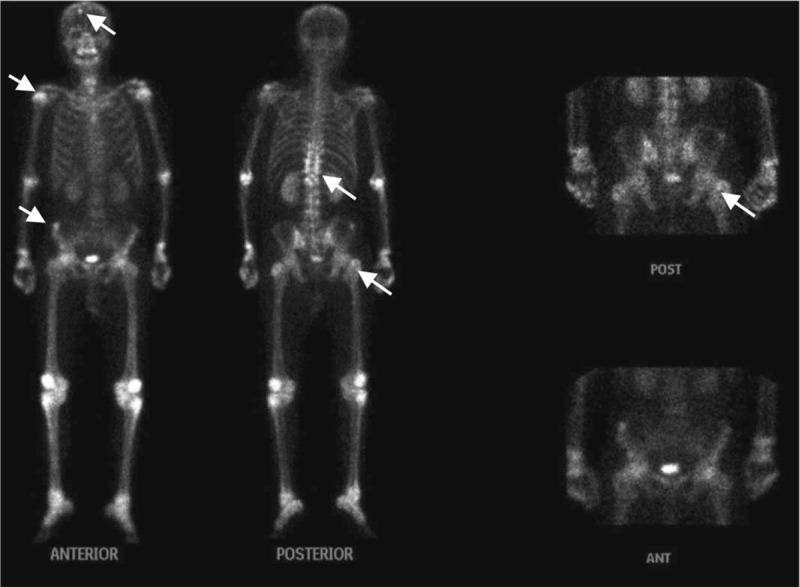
^99m^Tc-MDP bone scintigraphy showed multiple lesions of increased activity in spine, pelvis, skull, scapula and humerus.
